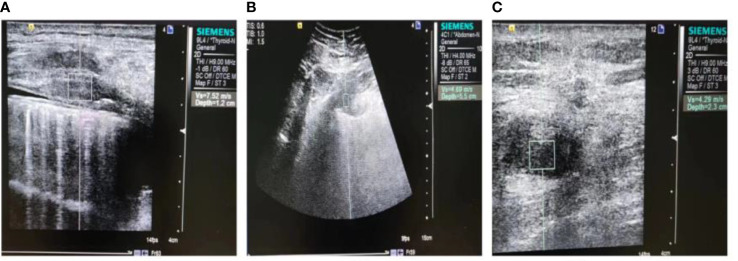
Ultrasound images of malignant chest wall and peripheral non-small cell lung cancers. (A) A solid hypoechoic nodule is displayed, with poorly defined borders and an irregular shape. The measurement depth is 1.2 cm and the VTQ value is 7.52 m/s. (B) A heterogeneous hypoechoic mass is shown, with unclear boundaries and an irregular shape. The measurement depth is 5.5 cm and the VTQ value is 4.69 m/s. (C) Another heterogeneous hypoechoic mass is displayed, with unclear borders, an irregular shape, and a burr-like appearance. The measurement depth is 2.3 cm and the VTQ value is 4.29 m/s.
